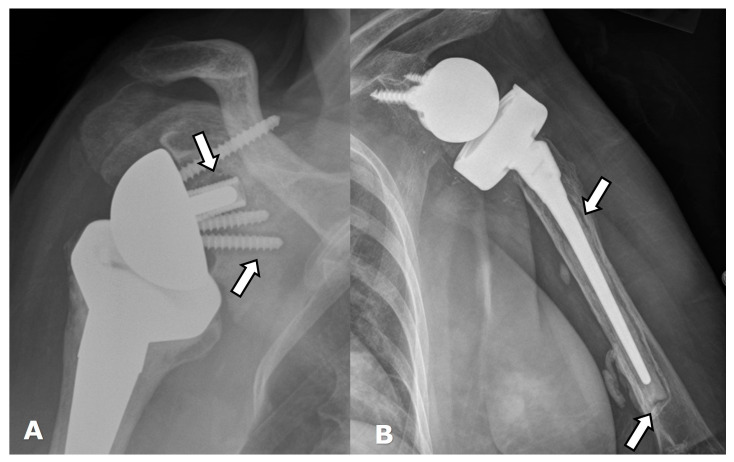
(A) Frontal radiograph in a patient with an RTSA shows lucency (arrows) along the baseplate screws and central screw consistent with loosening. (B). Frontal radiograph in a different patient with RTSA shows lucency (arrows) around the humeral component as well as the bone–cement interface, consistent with loosening. Cortical thinning is also noted along the medial aspect of the distal humeral stem.
